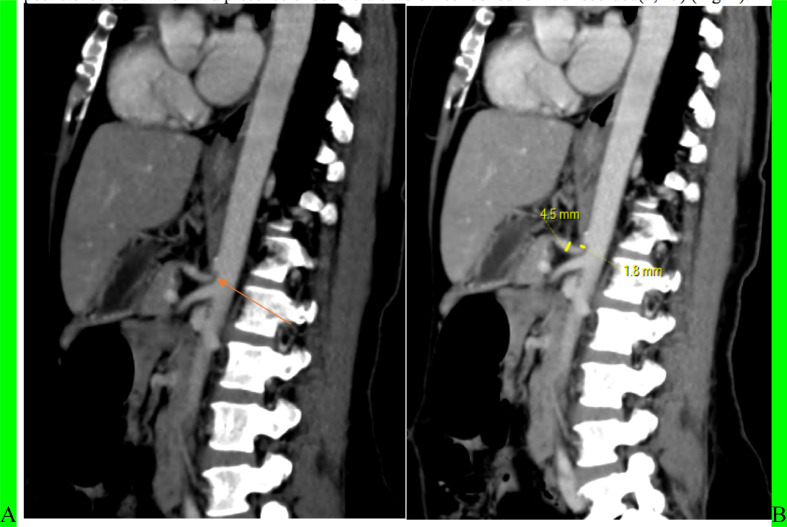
Abdominopelvic CT of 60-year-old, known cervical ca patient. This mid sagittal image shows the celiac artery is compressed by median arcuate ligament (arrow) (A) and has hooked appearance and narrowing (B).
